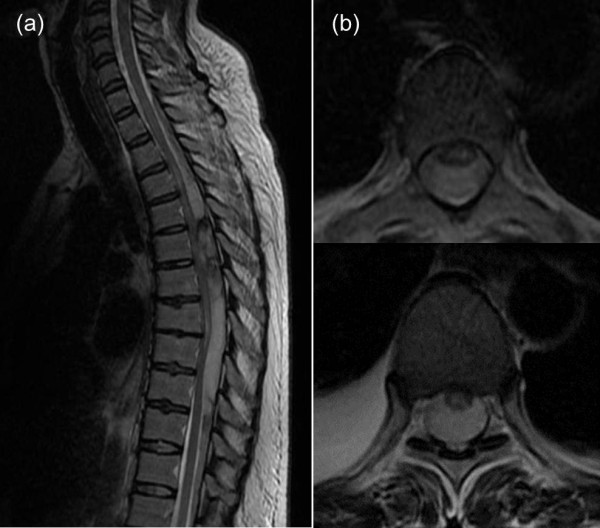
T2-weighted thoracolumbar MRI scan images obtained immediately after Cesarean section. (a) Sagittal plane. (b) Coronal plane. A space-occupying lesion showing signal hyperintensity is apparent in the upper spinal canal at the level of the second to ninth thoracic vertebrae.
